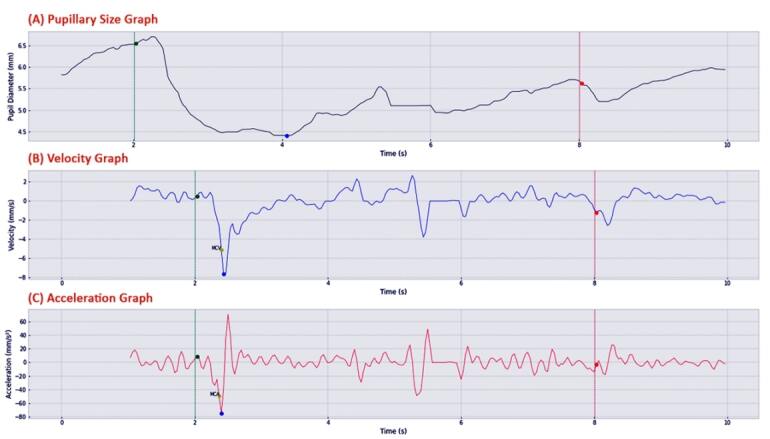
The measurement of pupillary response parameters using the ALZEYEMER software resulted in three graphs. The measurement graph starts and ends as the IR-LED lights are up and off for ten seconds. The green line indicates the beginning of the white LED light intervention, which lasts six seconds, and the red line indicates the end of the intervention. (A) The pupillary size graph shows D1 (green dot), D2 (blue dot), and the difference between D2 and D1 is calculated as ΔD. The range between the green line and the green dot is LT, while the range between the green dot and the blue dot is CT. (B) The velocity graph shows MCV (yellow dot), and ACV is calculated based on average velocity data between the green dot and the blue dot. (C) The acceleration graph shows MCA (yellow dot), and ACA is calculated based on average acceleration data between the green dot and the blue dot.
